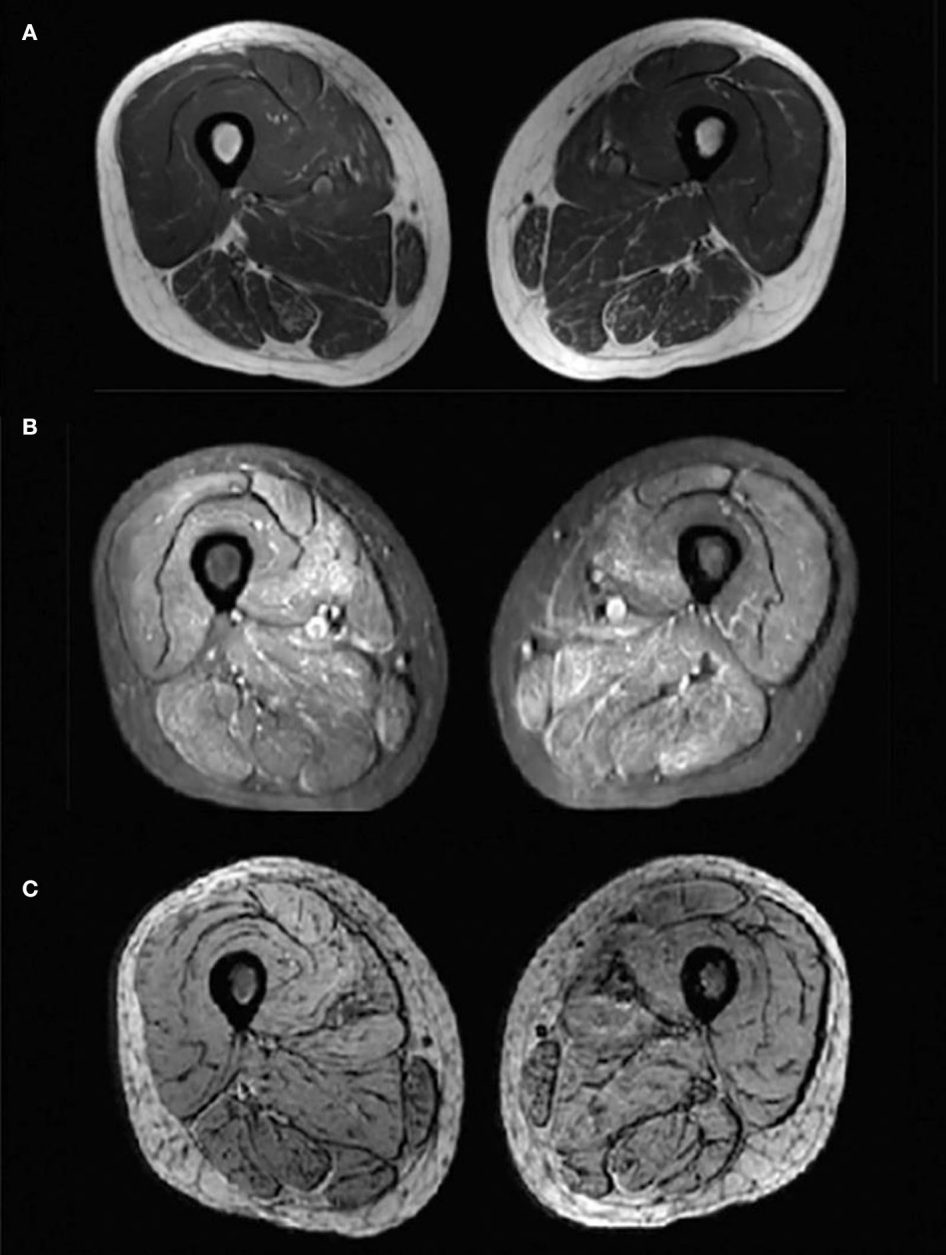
Magnetic resonance imaging (MRI) changes of bilateral thigh. Axial-T1-weighted MRI (A) showed hypointensity indicating no obvious fatty infiltration of the muscles and axial STIR MRI (B) showed slightly hyperintensities indicating mild muscle oedema. No hypointense structure was observed on axial SWI MRI (C), indicating no angiogenesis.
